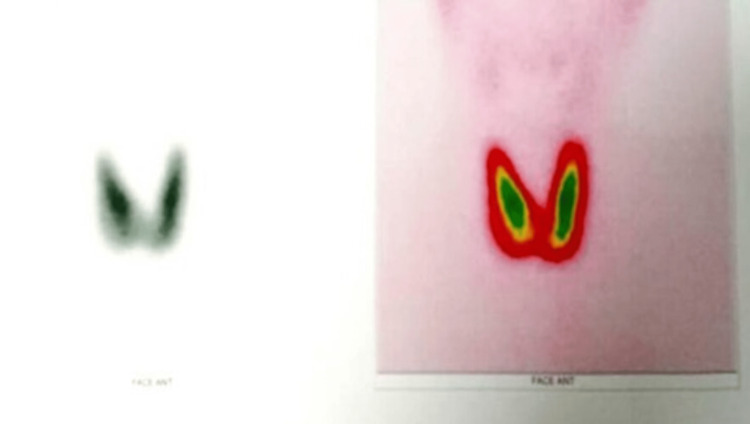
Acquisition of a static anterior image centered on the cervical region, 20 minutes after injection of 5 mCi of 99 mT Thyroid scintigraphy demonstrating an enlarged gland with diffuse, homogeneous, and markedly increased tracer uptake, consistent with Graves’ disease.
